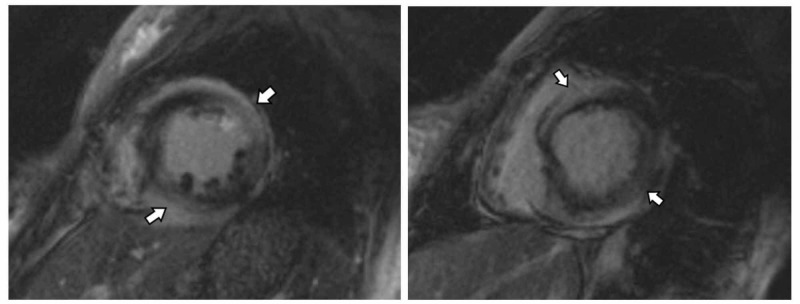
Cardiac MRI in the short axis views demonstrate abnormal delayed gadolinium enhancement (arrows) involving mid-myocardial and sub-epicardial regions of multiple segments of the left ventricle. MRI: Magnetic resonance imaging.
